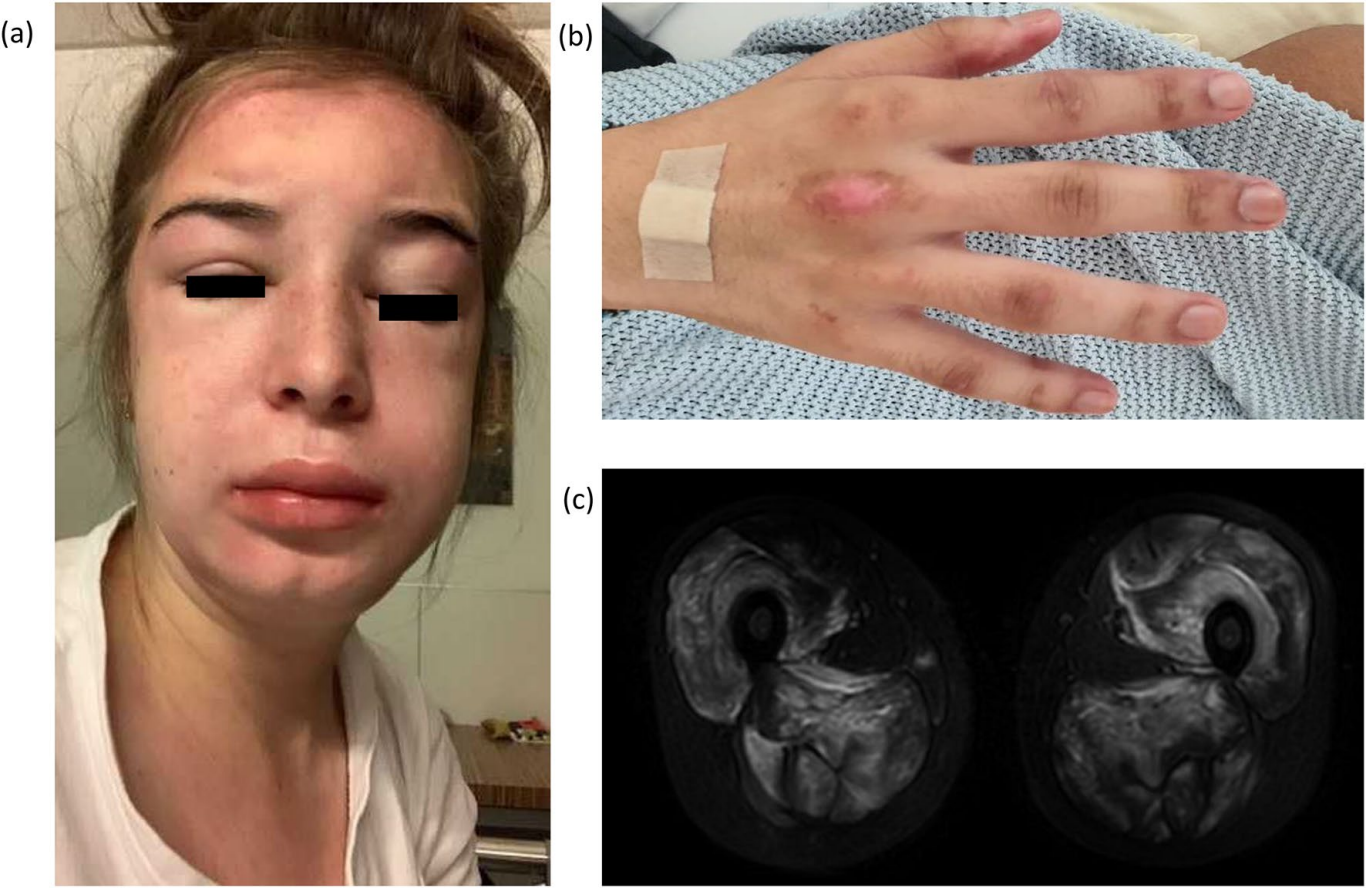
Patients’ images: a Patient 2: facial swelling, heliotrope erythema. b Patient 1: Gottron papules c Patient 2: magnetic resonance imaging scan (T2) showing bilateral active myositis in the adductors and extensors of the thighs
